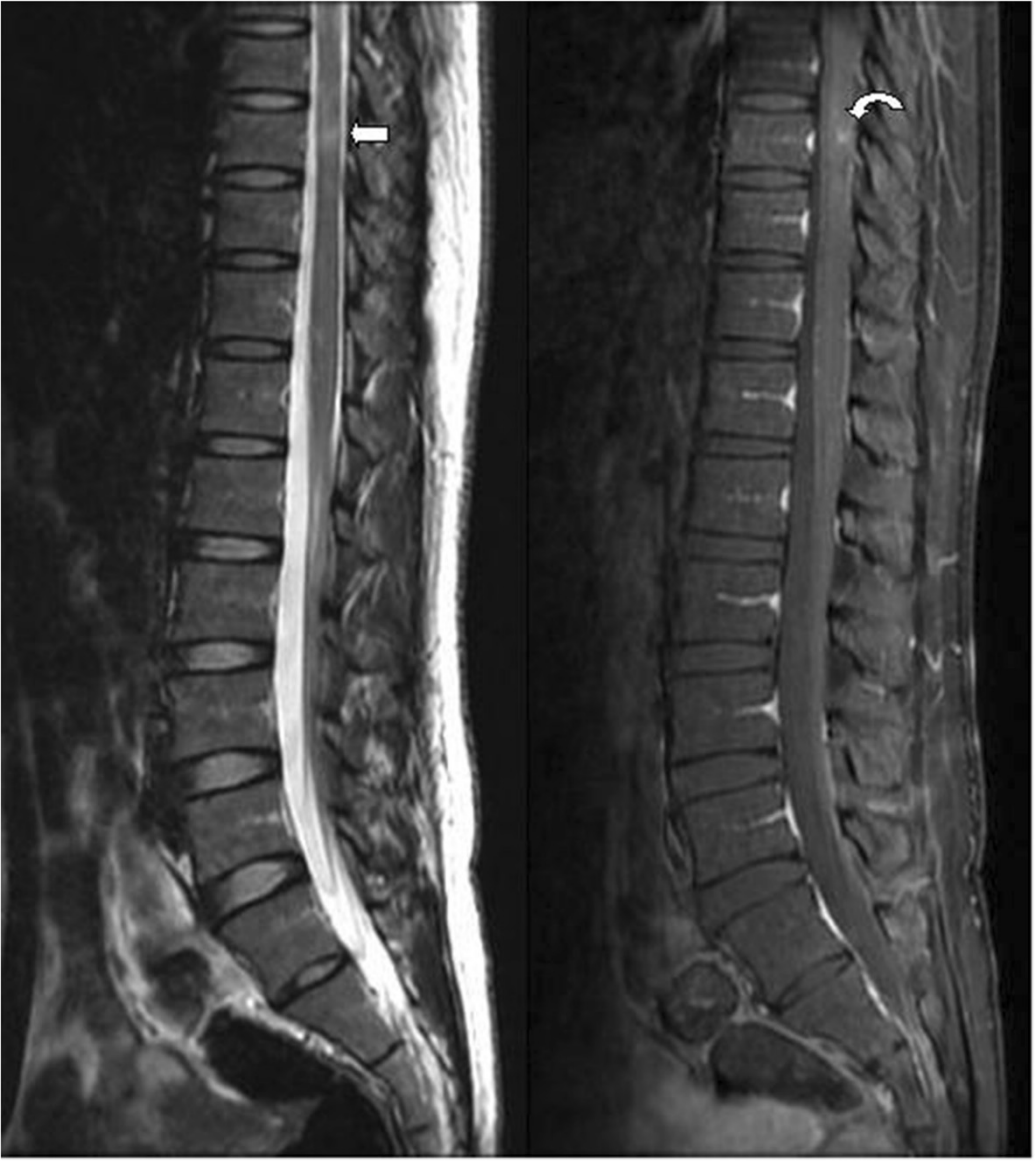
Low thoracic and lumbar spine MRI showing a hyperintense lesion in the spinal cord (T9 level), which is observable on the T2-weighted image (arrow). The lesion exhibits contrast enhancement after gadolinium injection (curved arrow).
